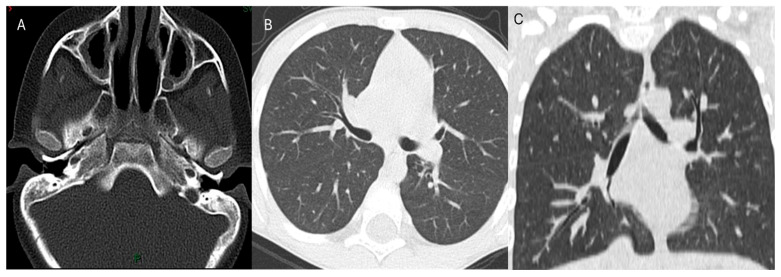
(A) Paranasal sinus CT: Axial CT demonstrates bilateral maxillary sinus mucosal thickening without a definite air–fluid level or bony erosion, consistent with chronic rhinosinusitis. (B,C) Chest CT (axial and coronal lung windows) shows no focal consolidation or pleural effusion. Coronal images show chronic airway changes with cylindrical bronchiectasis.
